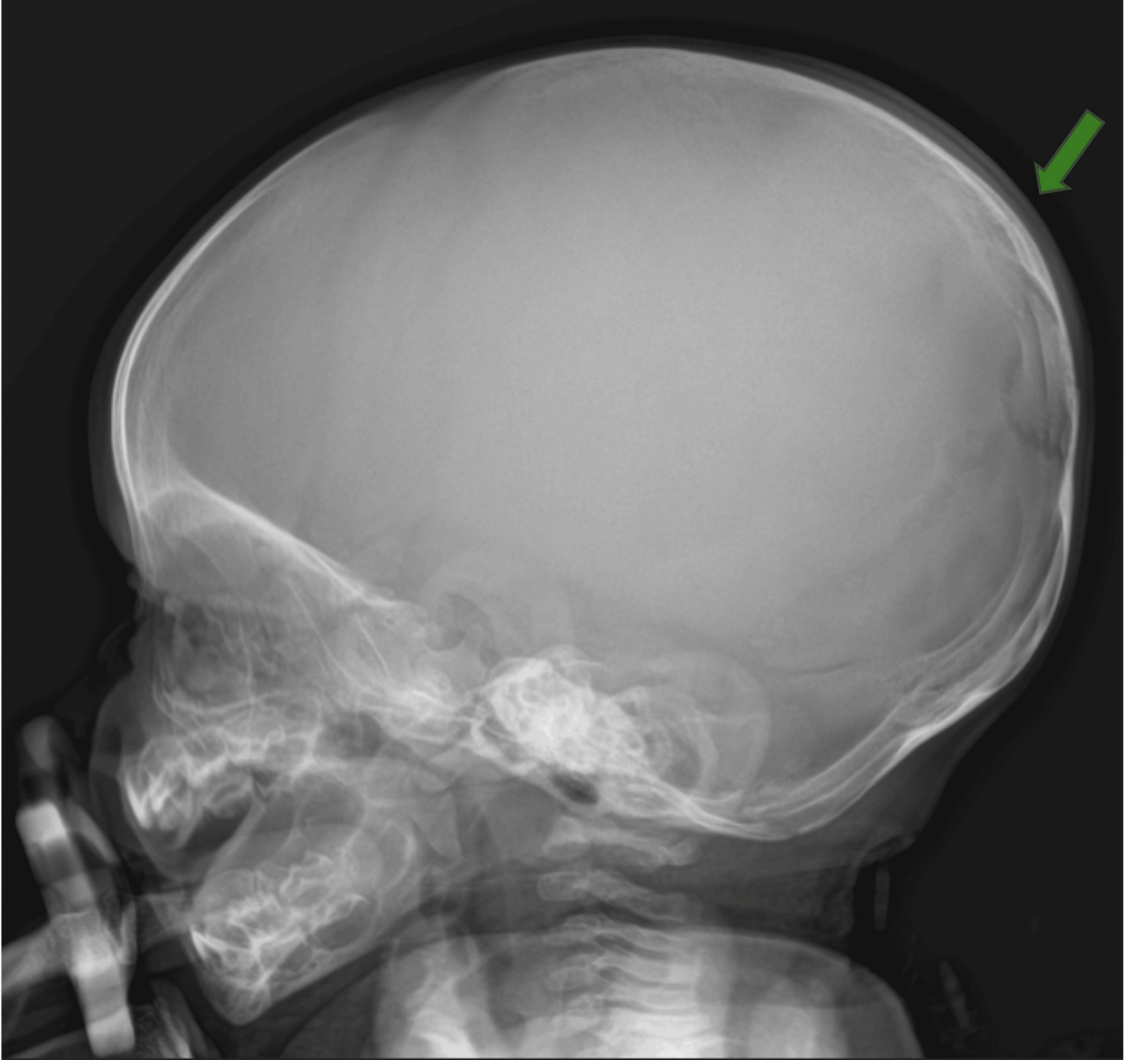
Cranial radiography performed in the emergency department shows no signs of bone fractures or masses. Green arrow: occipitoparietal region without evidence of bone injury.
